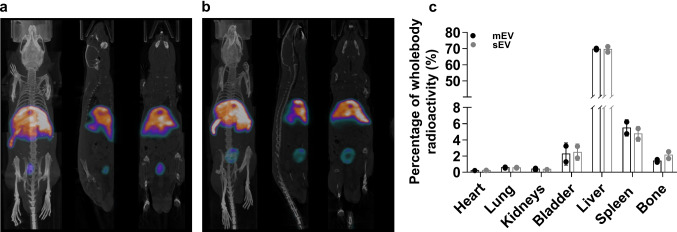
In vivo SPECT/CT images of the distribution of ^99m^Tc-HYNIC-Duramycin-labeled HEK293T-palmGFP sEV (a) and mEVs (b). The 3D reconstructed and co-registered SPECT and CT images are shown together with sagittal and coronal images (from left to right) (a and b). Quantitative distribution of sEV and mEV samples (c). Activities are measured in the specified organs as a percentage of the wholebody radioactivity
